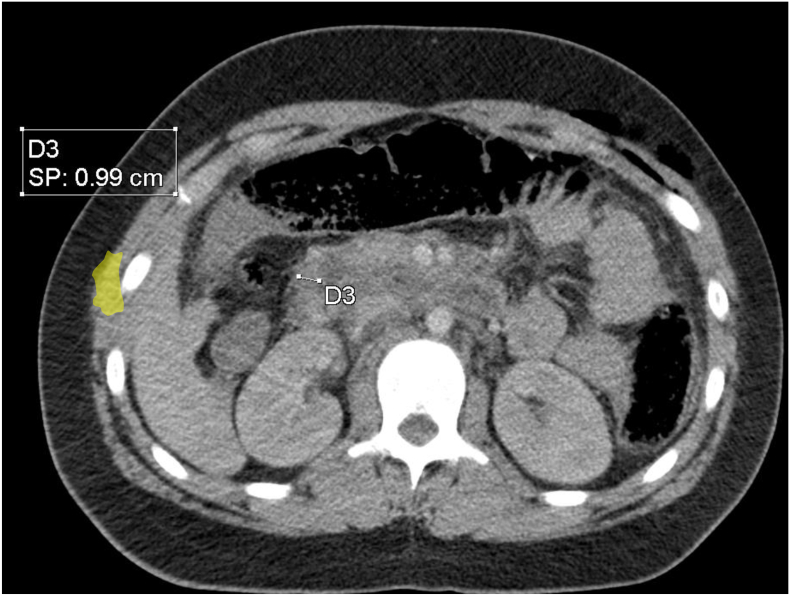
Axial computed tomographic section demonstrating circumferential wall thickening of the duodenum on his third portion (D3).
